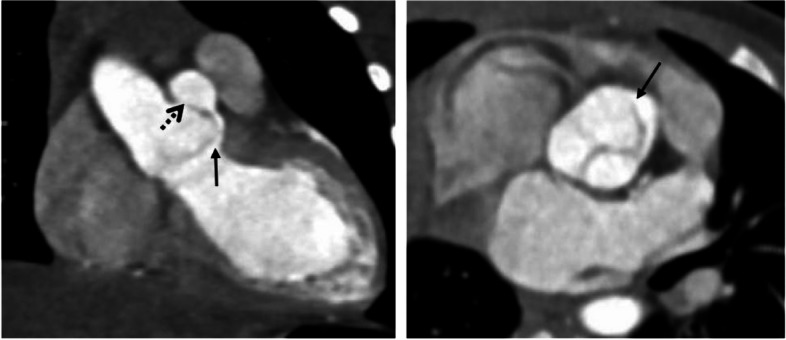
Cardiac computed tomography. Aortico-left ventricular tunnel originating from sinotubular junction (dotted arrow) above right coronary sinus, running lateral side of right coronary cusp, and communicating with left ventricle beneath left–right coronary commissure (bold arrow)
